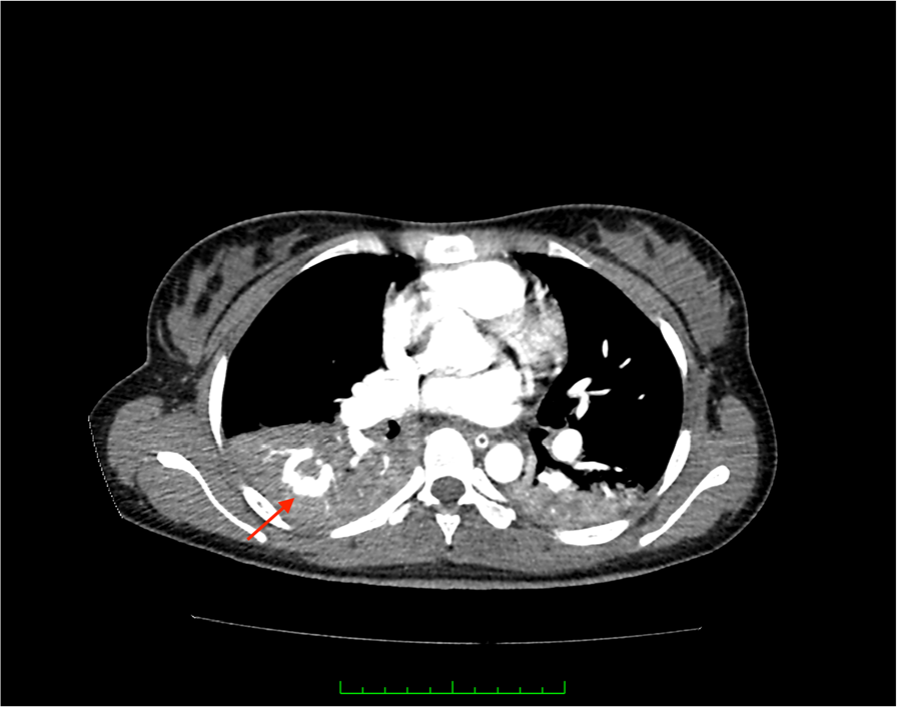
CT angiography of the thorax, in axial section showing presence of a pulmonary AVM (red arrow). Note also presence of hemothorax.
